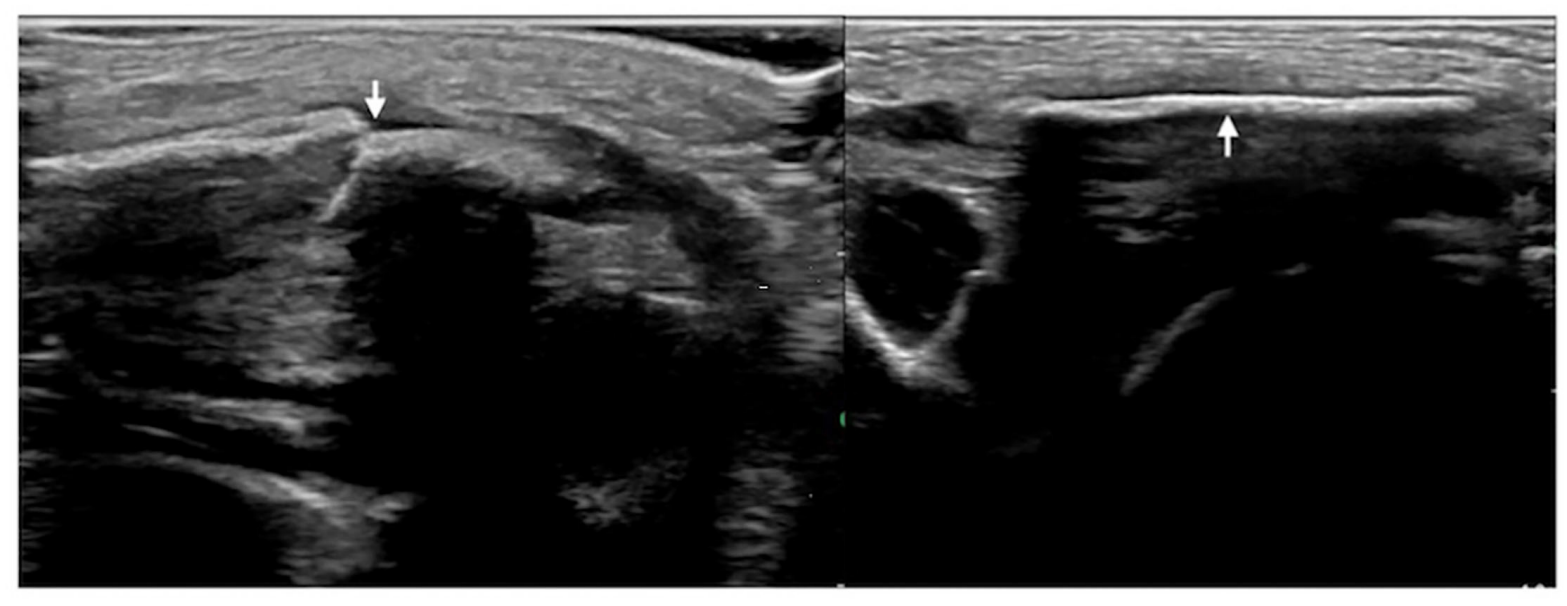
Left clavicle fracture. The infant was G_4_P_2_ with a gestational age of 41 weeks, forceps delivery, and a birth weight of 3,670 g. The patient was admitted to the hospital 41 h after birth due to jaundice for 6 h. Physical examination revealed asymmetrical clavicles as well as local swelling and bone rubbing on the left side. Ultrasound showed interrupted cortical continuity, broken end formation, and dislocation in the left midclavicular segment (left); the right clavicular continuity was intact, and the cortical bone was smooth.
